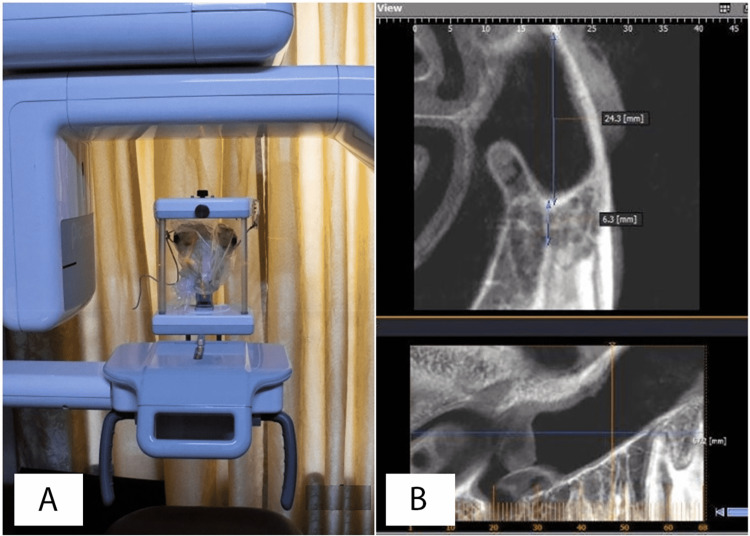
A: The CBCT scan using Picasso® Pro CBCT system. B: The determination of the appropriate location of elevation CBCT: cone-beam computed tomography
